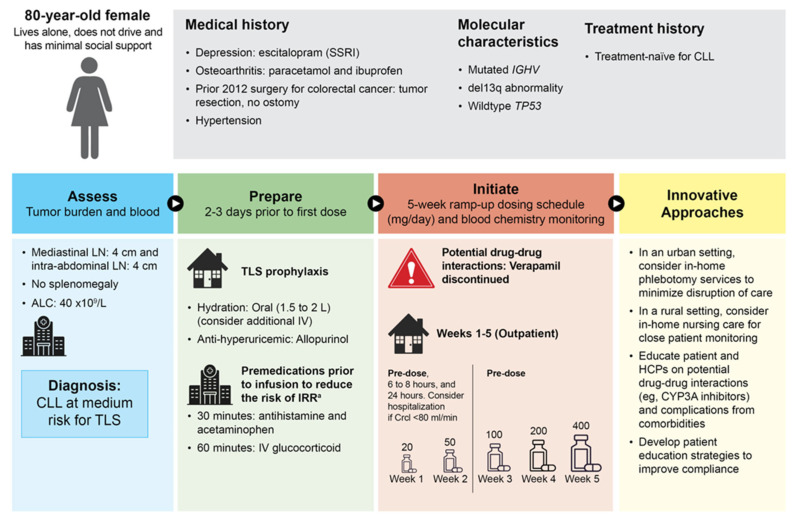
Patient case 2: medium risk for tumor lysis syndrome (treatment-naïve patient treated with fixed-duration venetoclax + obinutuzumab). ^a^ Some institutions will give corticosteroids (e.g., dexamethasone, 20 mg) and antihistamines (e.g., diphenhydramine, 25 mg) the night before and morning of the first obinutuzumab infusion, in addition to giving premedications right before the infusion. ALC, absolute lymphocyte count; CLL, chronic lymphocytic leukemia; CrCl, creatinine clearance; CT, computerized tomography; CYP3A, cytochrome P4503A; HCP, healthcare provider; IGHV, immunoglobulin heavy variable; IV, intravenous; IRR, infusion-related reaction; L, liter; LN, lymph node; mg, milligram; SSRI, selective serotonin reuptake inhibitor; TLS, tumor lysis syndrome.
